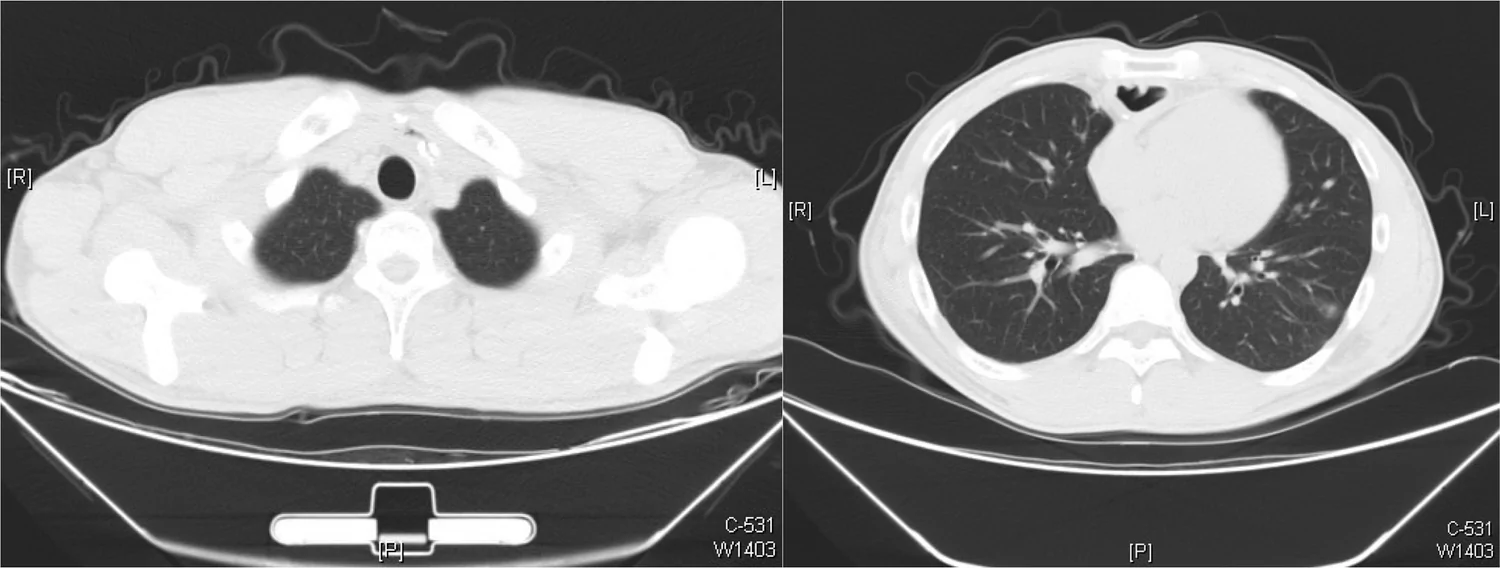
Follow-up chest CT after Stage II surgery showing complete resolution of pleural effusion and pneumomediastinum, well-expanded lungs, and visualisation of the retrosternal tubular gastric conduit.
